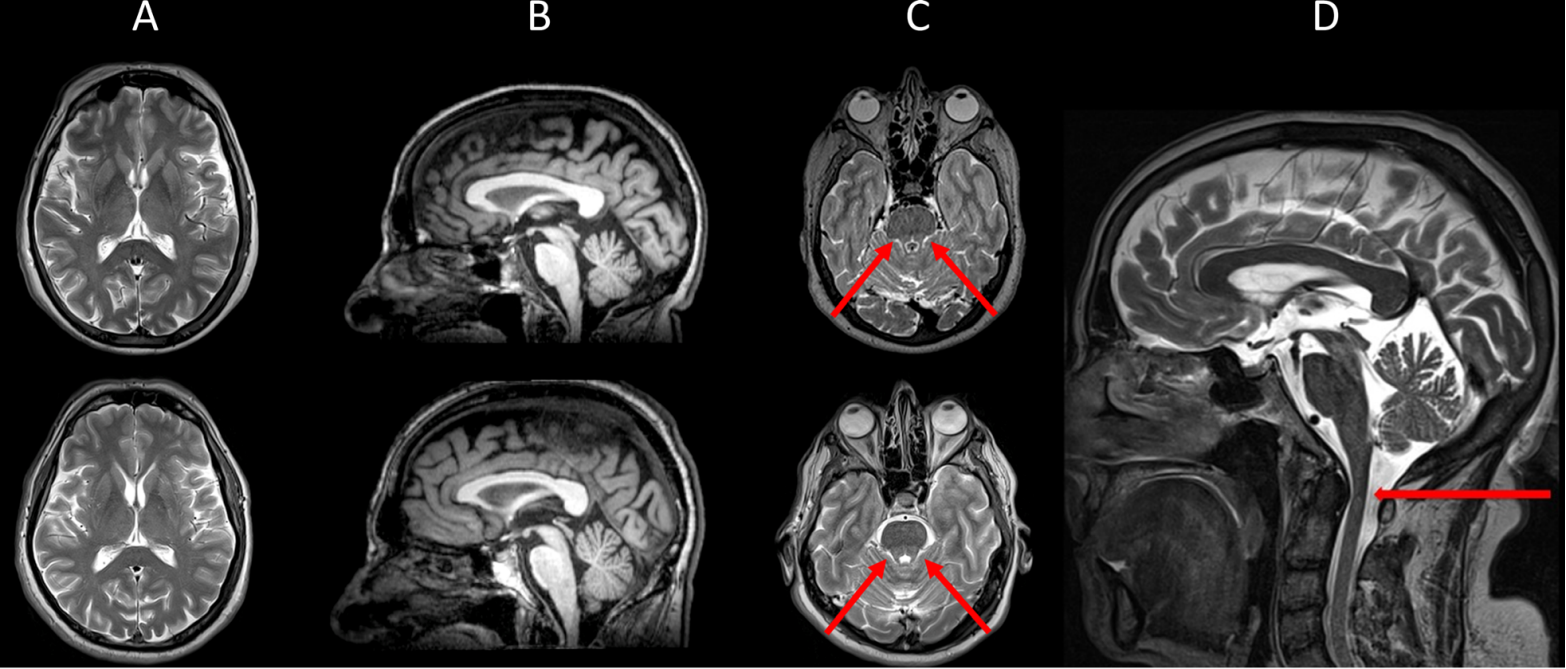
Brain MRI examination showing findings in patient III3 (upper row) and patient III 4 (lower row). Representative axial T2‐weighted (A, C) and reformatted sagittal T1‐weighted (B) images show absence of supratentorial atrophy or signal alterations (A, B). T2‐weighted images showing mild hyperintensity affecting both superior cerebellar peduncles (red arrows in C) and a slight spinal cord cervical atrophy (red arrows in D).
